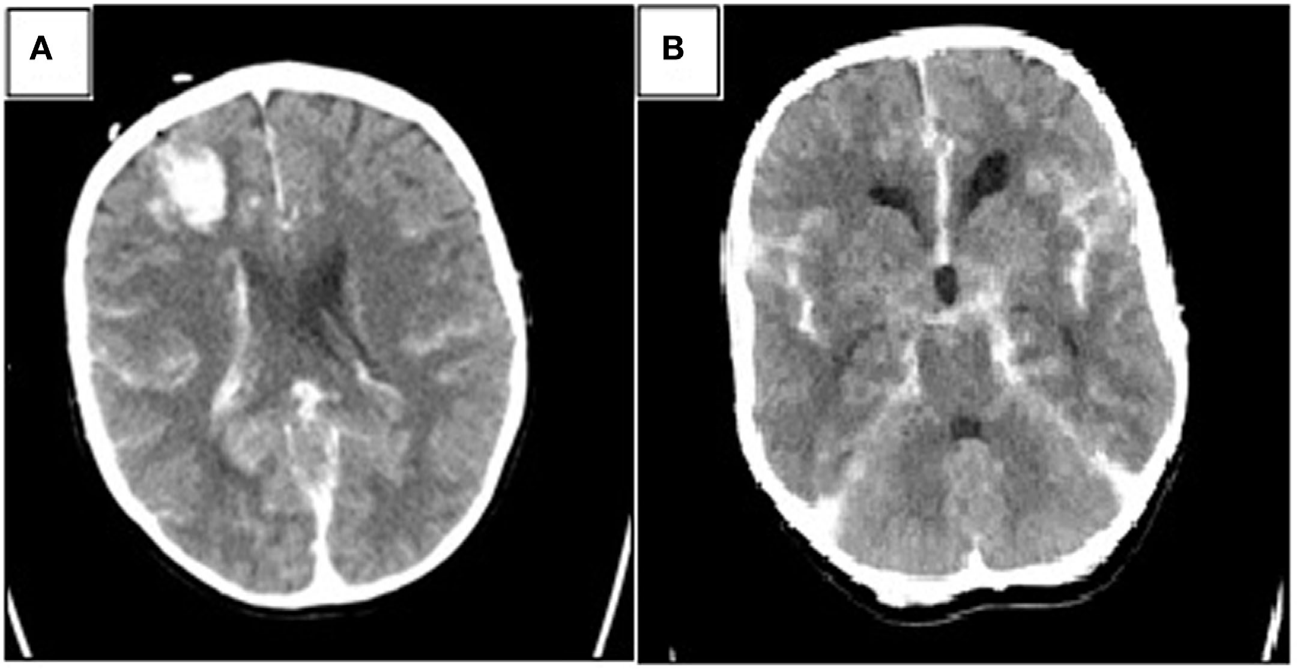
Postoperative head computed tomography (CT) showing (A) small amount of hemorrhage along the choroid plexus with a small hematoma in the frontal right lobe corresponding with the ventricular shunt tap and (B) basal cisterns with hemorrhage (Fisher 3).
